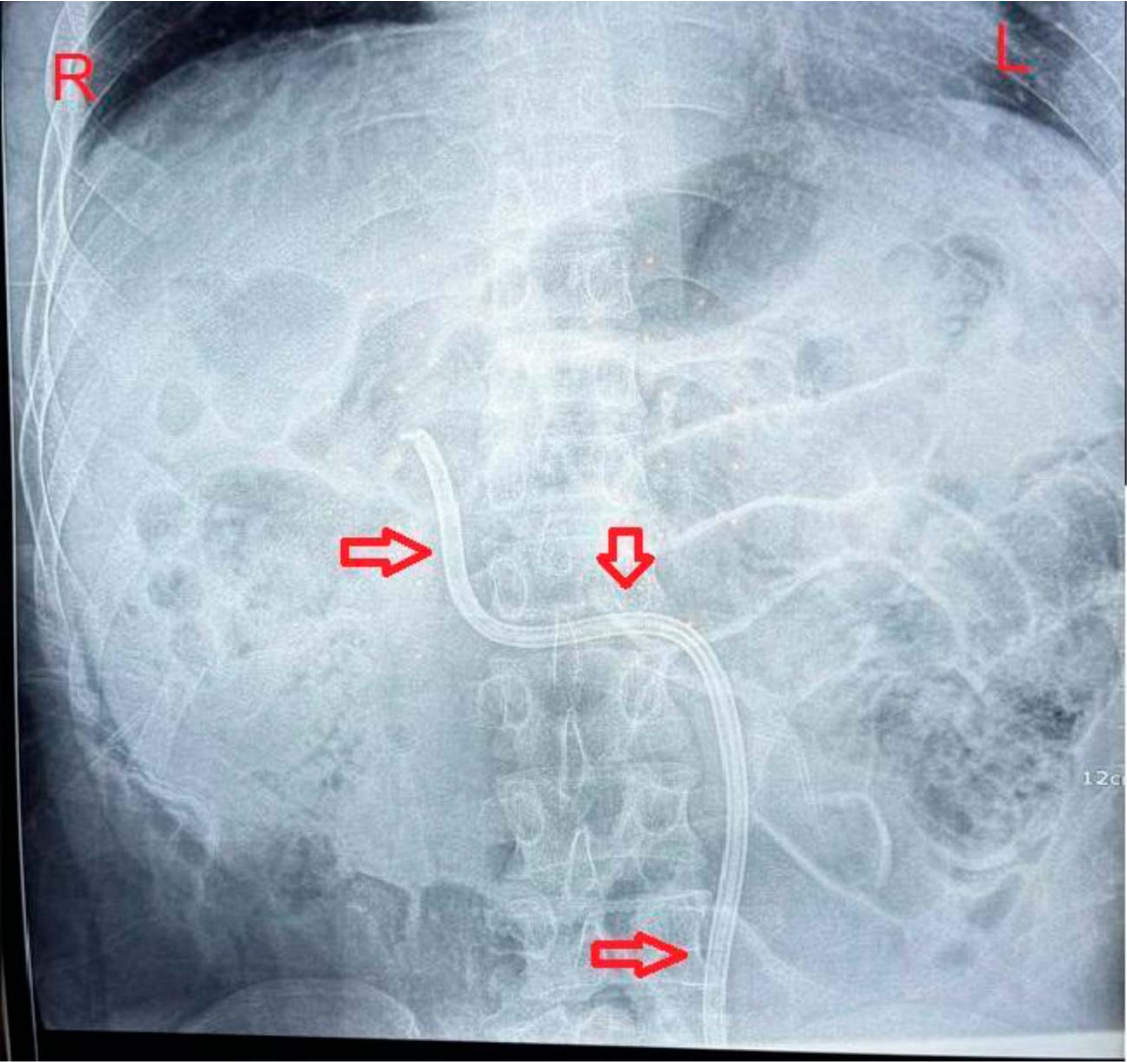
Abdominal radiograph of a tunneled venous catheter shows an atypical, serpiginous subcutaneous course ascending from the left inguinal region toward the upper abdomen. The catheter's tip is directed toward the right upper quadrant, likely terminating near the right suprahepatic vein. Red arrows highlight the path of the catheter along its intravascular trajectory.
